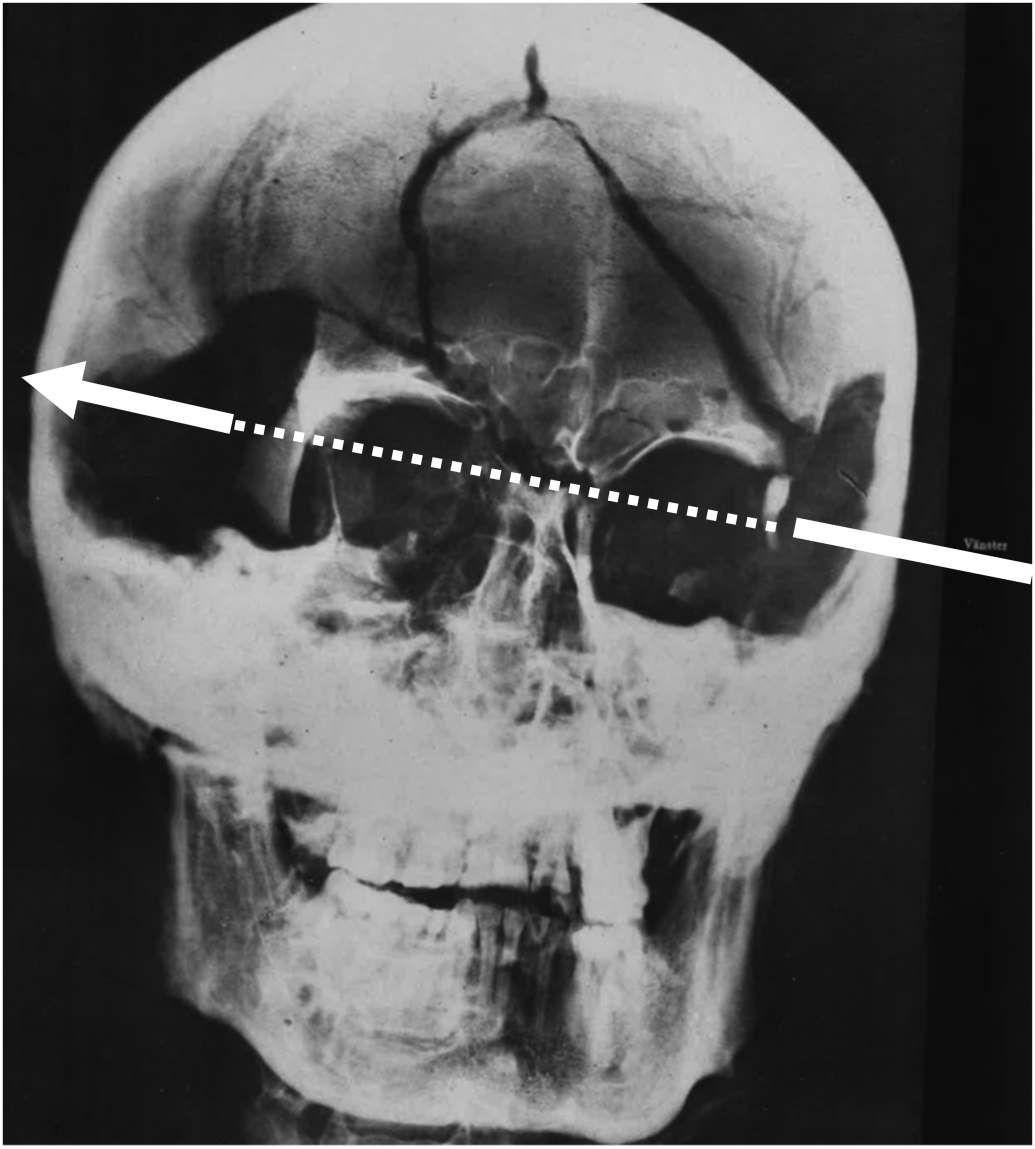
A radiograph from the latest autopsy (1) showing the wound channel and direction of the projectile.
